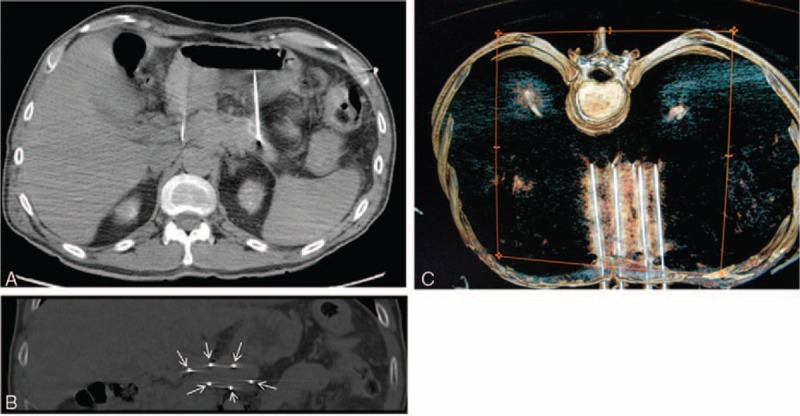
A, CT scan (axial view) showing 2 of the needles placed at the ends of the tumor. B, CT scan (coronal view) showing the 6 needles placed at the edge of the tumor (arrows). C, CT scan 3D (superior view) showing the 6 needles placed.
